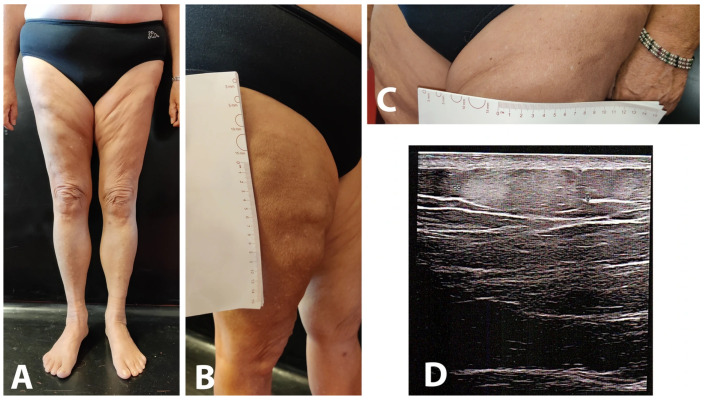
Lipomas in Pt. 2 at T30, following a 6 kg weight loss (A–C). Ultrasound imaging of a lipoma in the same patient (D).
